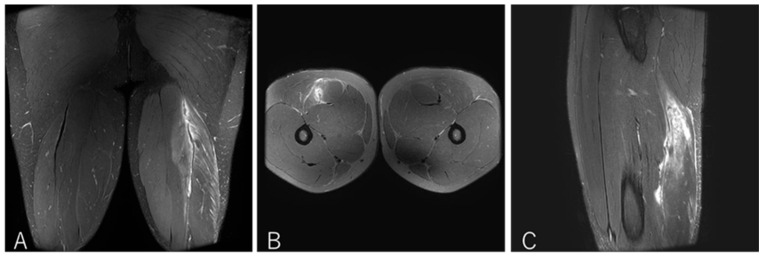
Case 3 MRI images: Conjoint tendon MTJ injury, Type I. (A) (Coronal image): The injured area was the proximal MTJ on CT, with haemorrhage observed bilaterally and tortuosity noted on CT. (B) (Axial image): prone position, bleeding is present on both BFLH and ST sides of the CT, and the CT is interrupted at the rupture site. (C) (Sagittal image): The CT is slightly retracted and accompanied by a haematoma.
